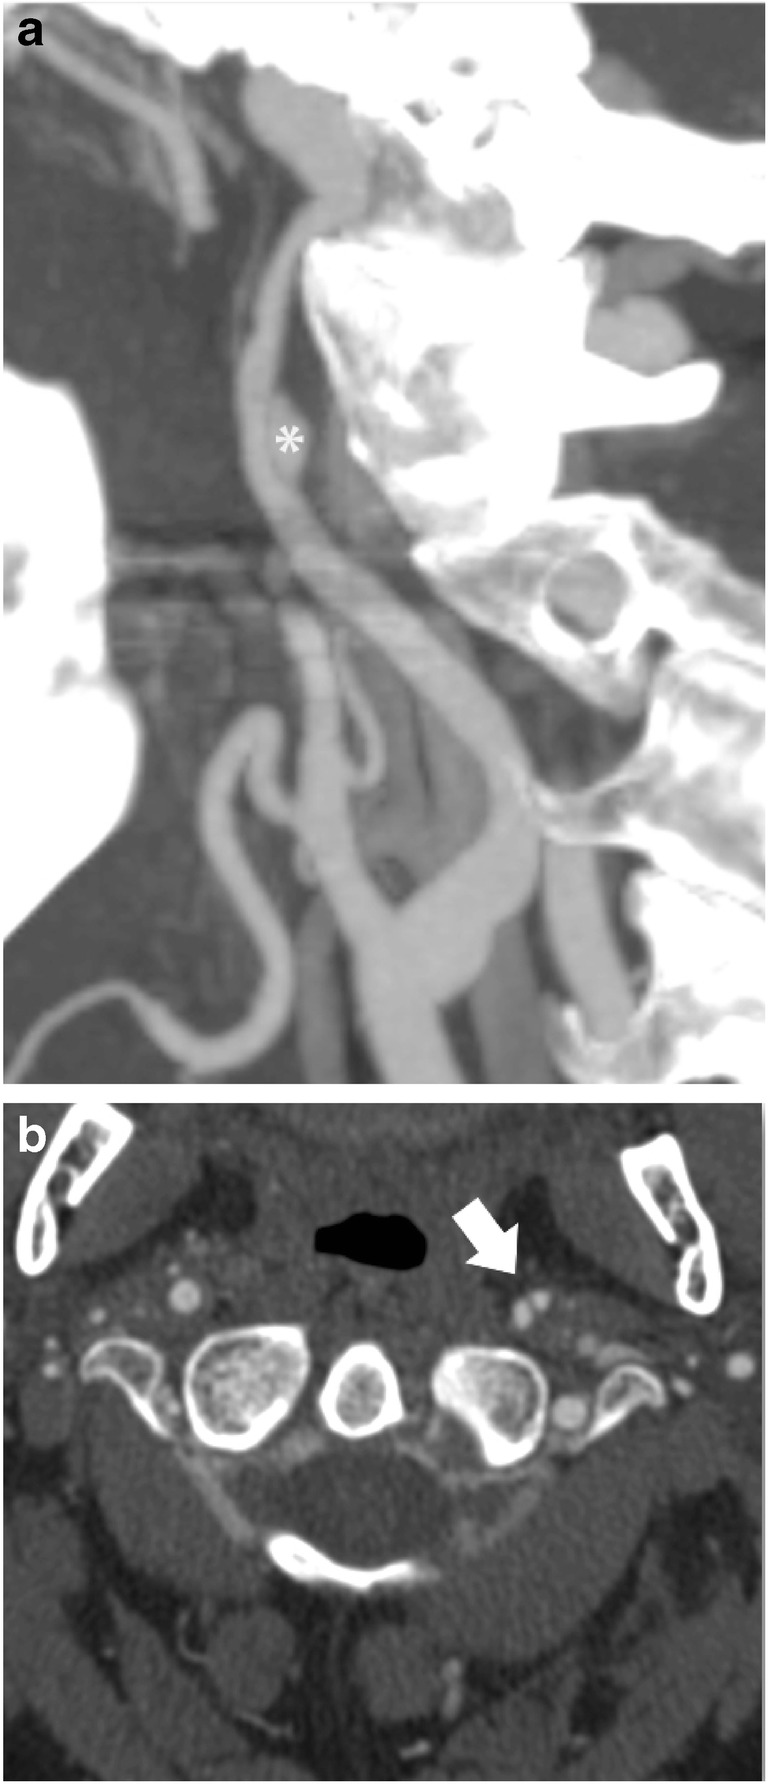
Grade III BCVI of the right ICA in a 51-year-old male after MVA. a Sagittal CTA MIP shows the outpouching of the lumen and arterial wall (asterisk) and b axial CTA images the double lumen indicating a pseudoaneurysm (arrow)
